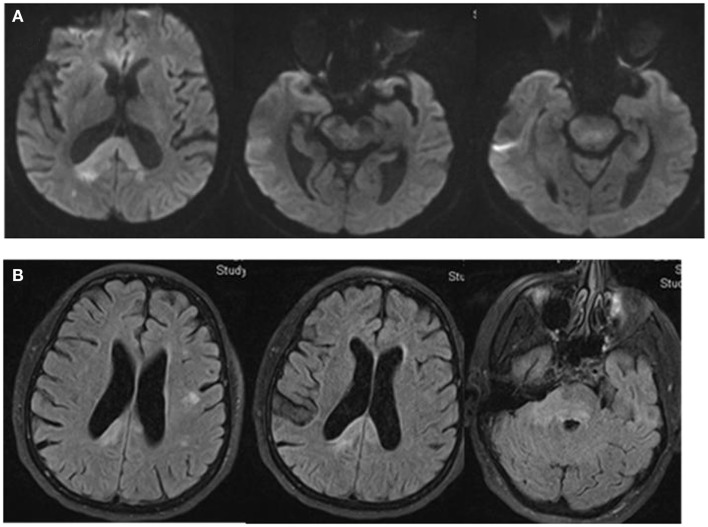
DW-MRI of the brain shows multiple areas of confluent restricted diffusion affecting the splenium of the corpus callosum, right medial occipital white matter, right brachium pontis, bilateral dorsal pons, and right cerebral peduncle (A). These areas are hyperintense on Fluid Attenuated Inversion Recovery (FLAIR) sequence. There is no evidence of mass effect (B).
